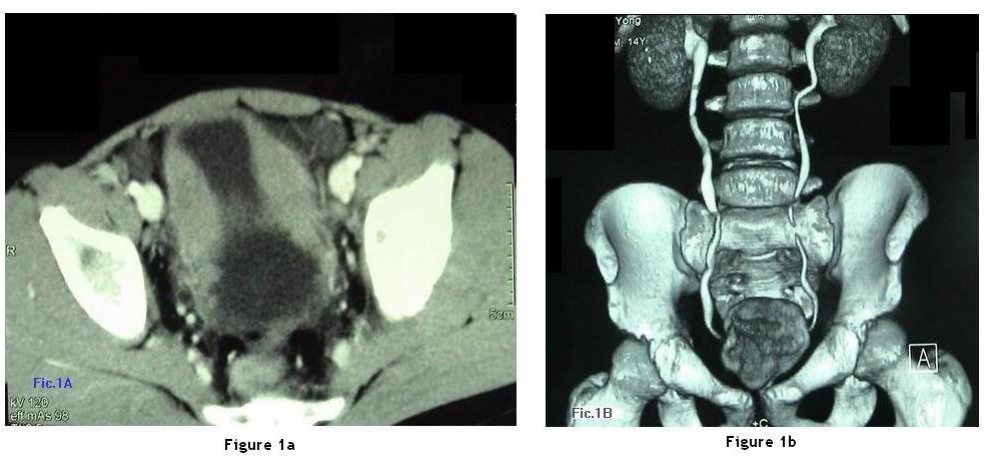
A. Computerized tomography of pelvis disclosed solid homogeneic-mass involving the wall of the dome and two lateral walls of the urinary bladder, average thickness of bladder wall was 1.9 cm. B. Computed tomography with three-dimensional imaging showed a normal upper urinary tract image with no nephrohydrosis, normal ureters and thickened walls of the bladder.
